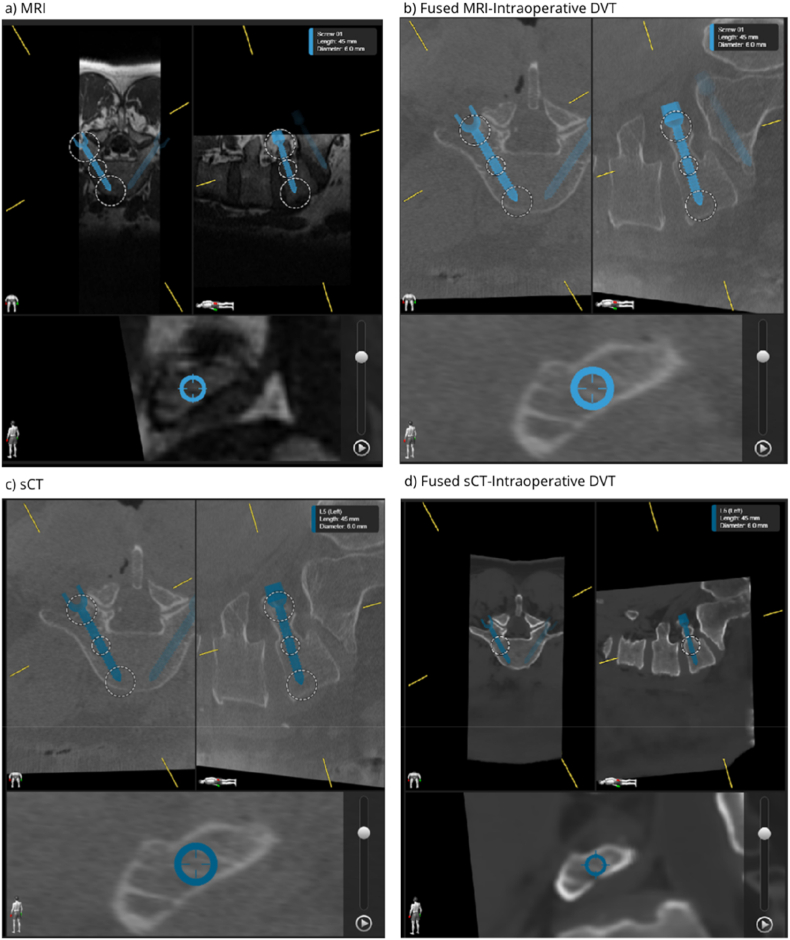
Example images for the different image modalities showing the transverse, sagittal, and trajectory view along the axis of a planned for the L5 vertebra in a single patient. a) MRI, b) fused MRI with intraoperative DVT, c) sCT, and d) fused sCT with intraoperative DVT.
